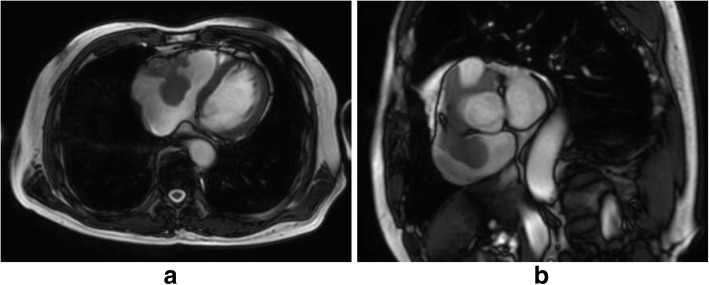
Preoperative cardiac MRI. Cardiac MRI showed a thickened anterior wall near the tricuspid valve with axial section (a) and a mass protruding into the lumen and expanding into the region between the aorta and pulmonary artery with coronal section (b)
